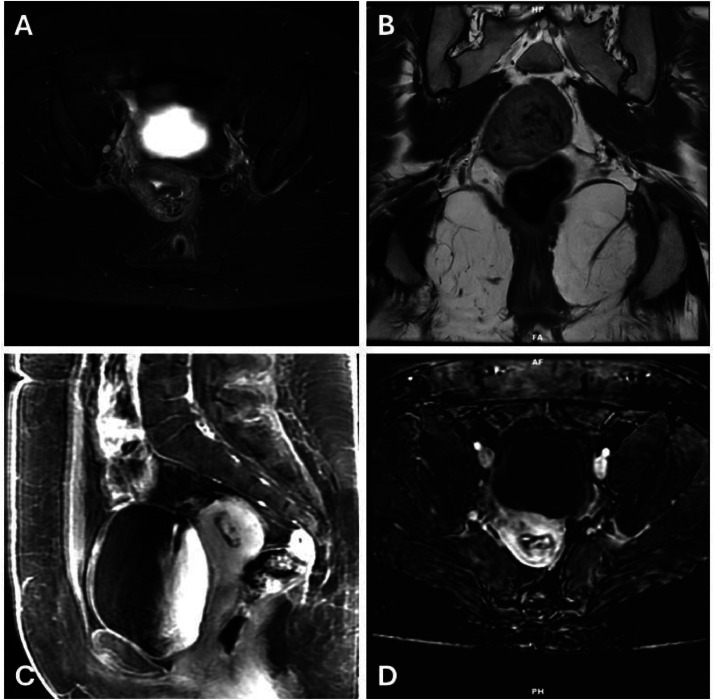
MRI – Axial Fat suppressed T2WI A), Coronal T2WI (B), Sagittal delayed postcontrast T1WI (C), and Axial Digital Subtraction Imaging (D). Features of serpiginous low T2 hypointense structures through the myometrium suggestive of myometrial vasculature pointing towards uterine AVM in concordance with hypervascular Doppler signal (A and B). Supporting this is the finding of incompletely maintained endometrialMyometrial interface on the posterior aspect (C). However, appearance of a high T1 signal mass in pre- and postcontrast images with enhancing components on subtraction imaging would favor the diagnosis of RPOC. Note: MRI, Magnetic resonance imaging; T2WI, T2-weighted imaging; T1WI, T1-weighted Imaging; AVM, Arteriovenous malformation; RPOC, Retained products of conception.
